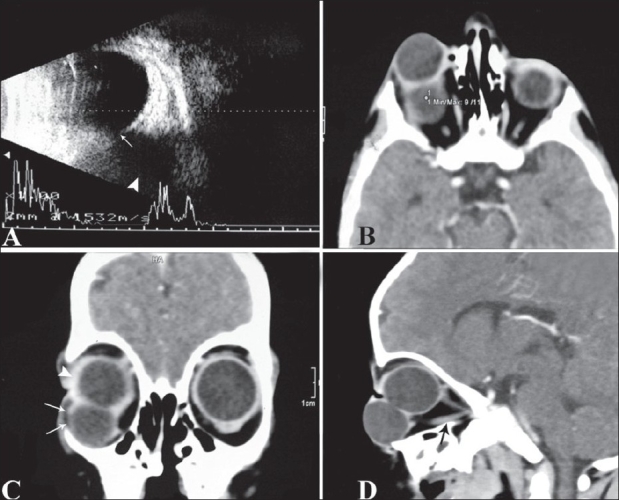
(A-D): B-mode USG (A) shows a well-defined cystic lesion (arrowhead) located in the inferior quadrant of the orbit with posterior acoustic enhancement, communicating with the globe (white arrow). Axial (B), coronal (C) and oblique sagittal multiplanar reconstruction (D) CT scan images show a colobomatous cyst on the right (arrows) with a normal-sized globe (arrowheads), the inferior rectus (black arrow in D) mimicking an optic nerve and giving rise to the appearance of a ‘double globe’
